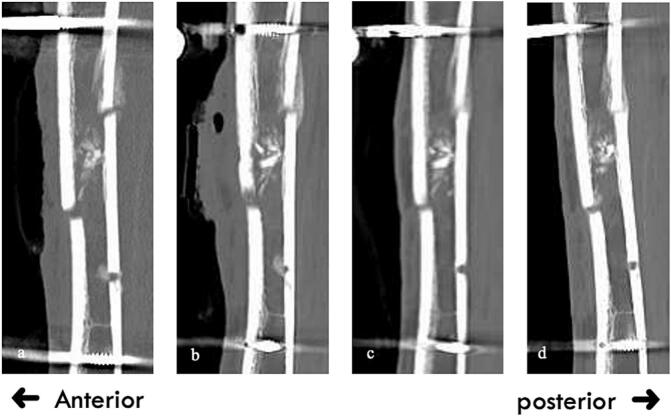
Sequential sagittal computed tomography images showing the healing process. a: 2 months after surgery. b: 3 months after surgery. c: 4 months after surgery. d: 5 months after surgery.
